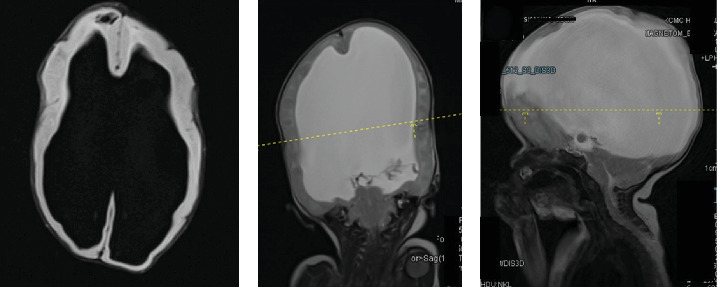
MRI scan of the brain revealed severe dilatation of the lateral ventricles, with thinning of brain parenchyma (A), the third and fourth ventricles appeared normal with effacement of aqueduct of Sylvius and tectal beaking, absent corpus callosum, small posterior fossa, and obliteration of cisterna magna. Bilateral clefts in the anterior frontal cortex, larger defect on the left measuring 0.7 cm in keeping with open lip schizencephaly (B, C).
